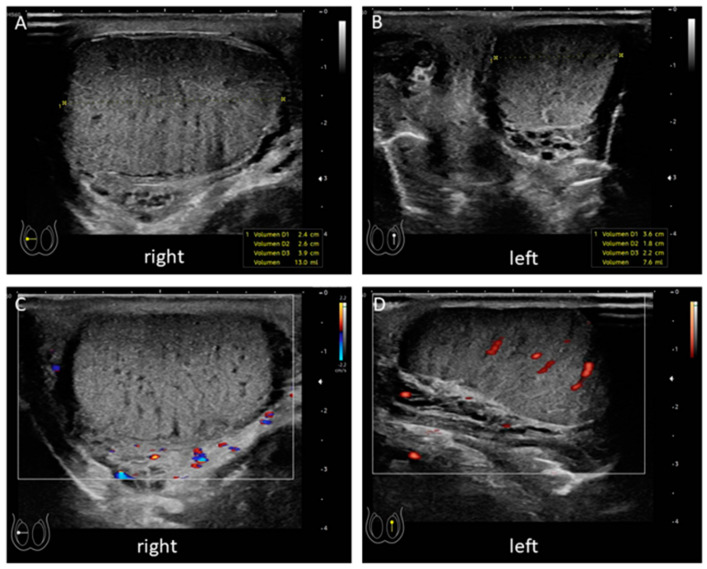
Point of care ultrasound. The initial ultrasound in the pediatric emergency room showed and enlarged the right testicle (A) compared to the left side (B). No perfusion of the right testicle could be detected (C) while the left testicle showed signs of central perfusion (D).
